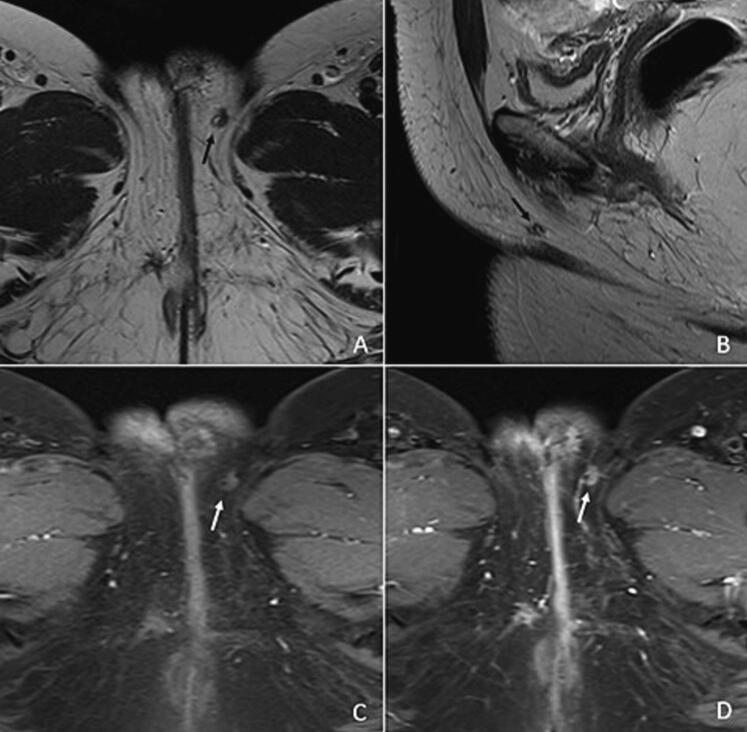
MR image of the superficial tumor recurrence. MR images show a focal well delimitated nodule located in the left labia majora, with a low signal on the T2 weighted-image (A and B), a spontaneous high signal on T1 fat sat weighted sequence (C), and a homogenous enhancement after contrast injection (D).
